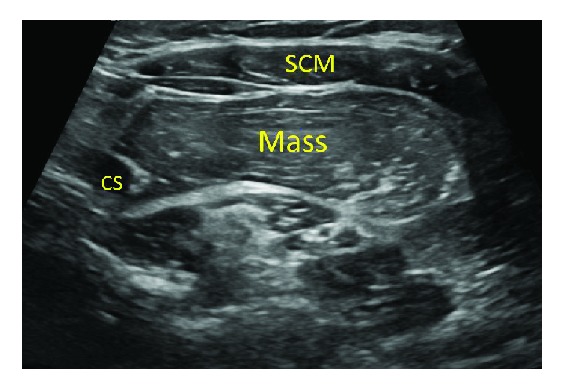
Ultrasound of the soft tissues of the left side of the neck in the area of clinical interest demonstrating a circumscribed solid echogenic mass measuring 6.7 cm × 1.8 cm × 4.8 cm consistent with a lipoma. The mass is displacing the sternocleidomastoid muscle (SCM) and carotid space (CS).
